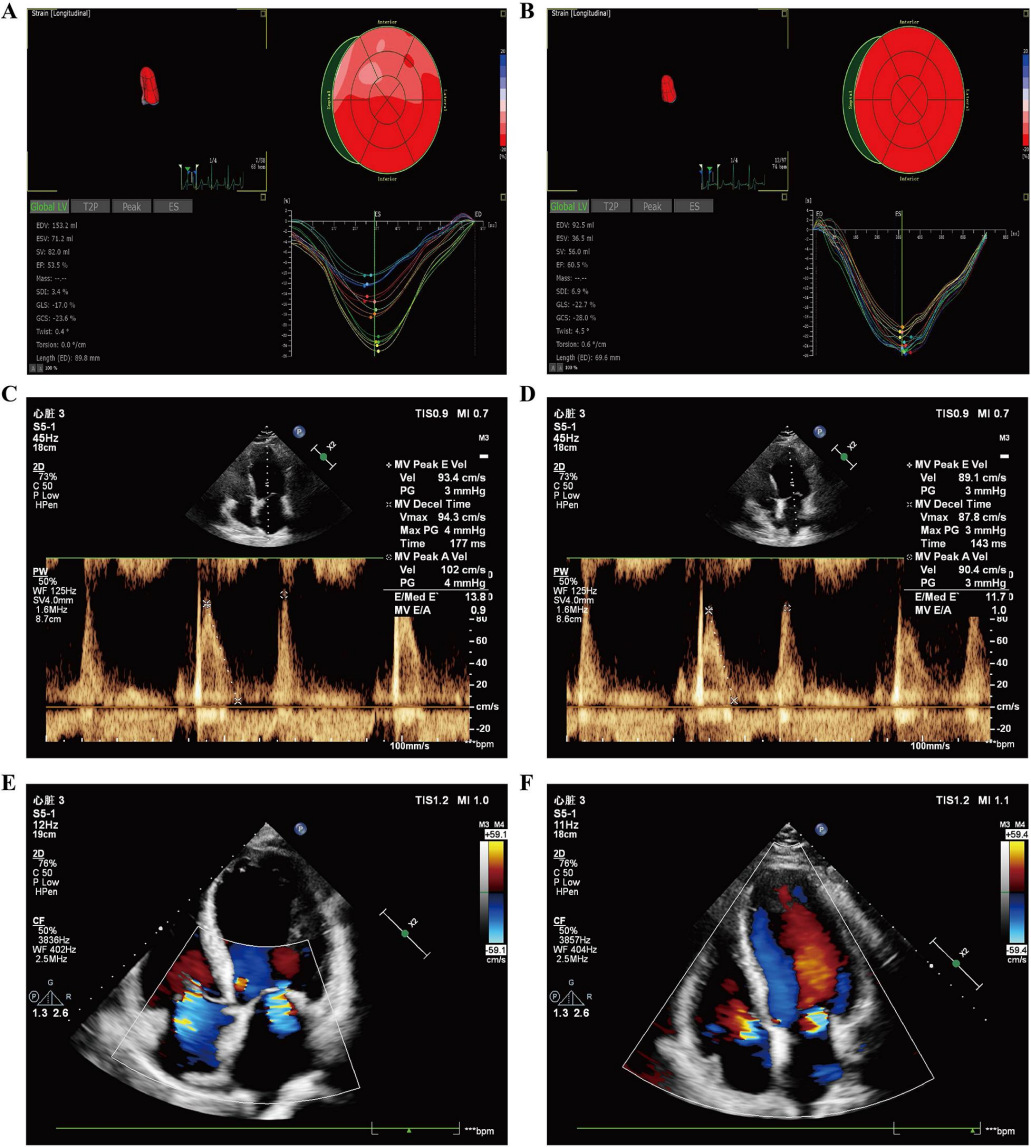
Left ventricular diastolic function and systolic function measured by three-dimensional speckle tracking echocardiography in UCM patients before and after renal transplantation. (A) Left ventricular three-dimensional volume, ejection fraction, strain parameters, and strain curves before renal transplantation. (B) Left ventricular three-dimensional volume, ejection fraction, strain parameters, and strain curves of the same patient after renal transplantation. (C) Left ventricular diastolic function before renal transplantation. (D) Improved left ventricular diastolic function of the same patient after renal transplantation. (E) Mitral regurgitation volume before renal transplantation. (F) Reduced mitral regurgitation volume of the same patient after renal transplantation.
